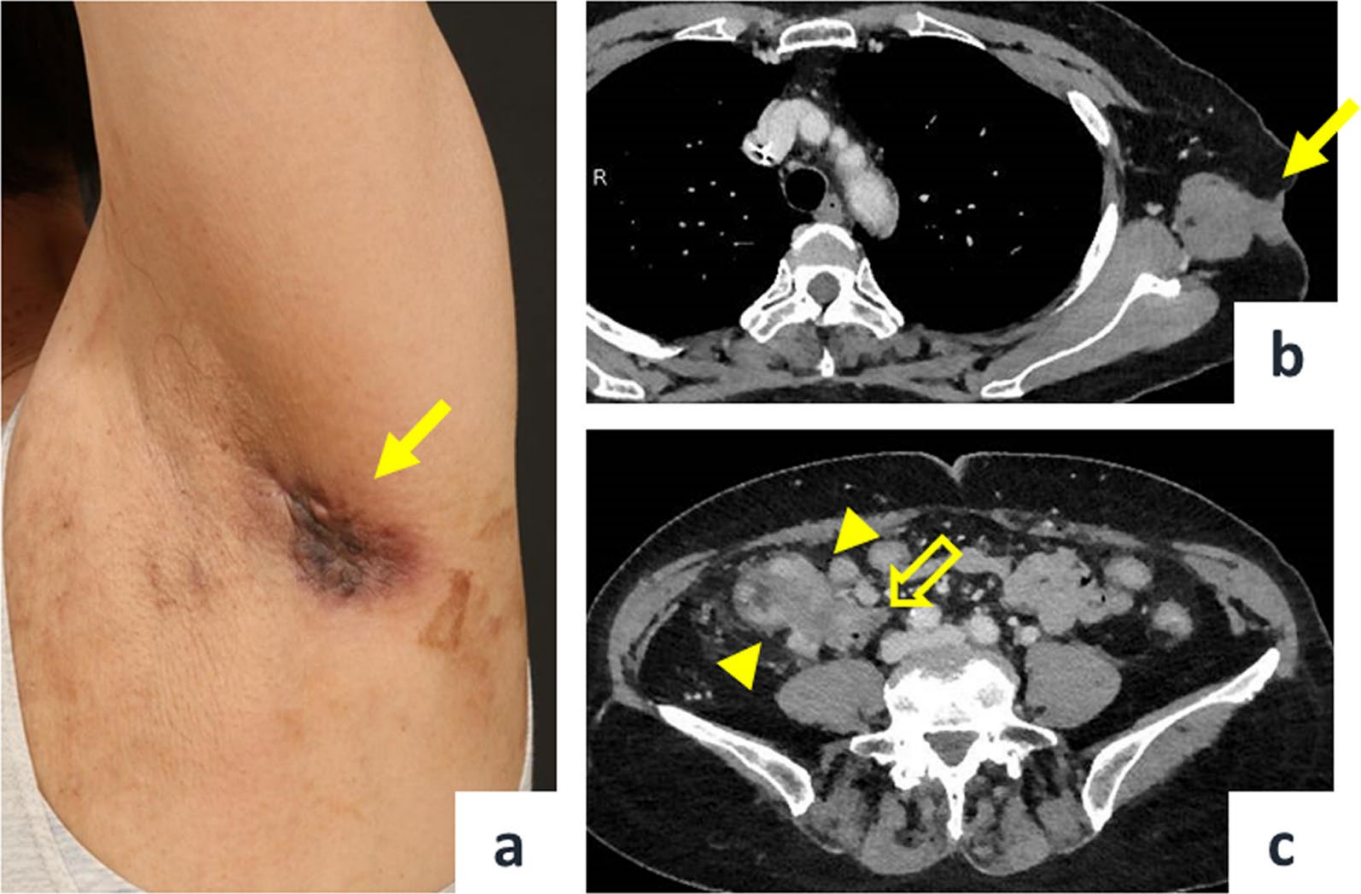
Post-chemotherapy clinical findings. a Axillary cutaneous tumor almost disappeared grossly (solid arrow). b CT scan of the chest shows the decreased size of the cutaneous tumor in the left axilla (solid arrow). c CT scan of the abdomen and pelvis shows the decreased size of the ascending colon cancer (arrowheads) with invasion of the duodenum (open arrow)
